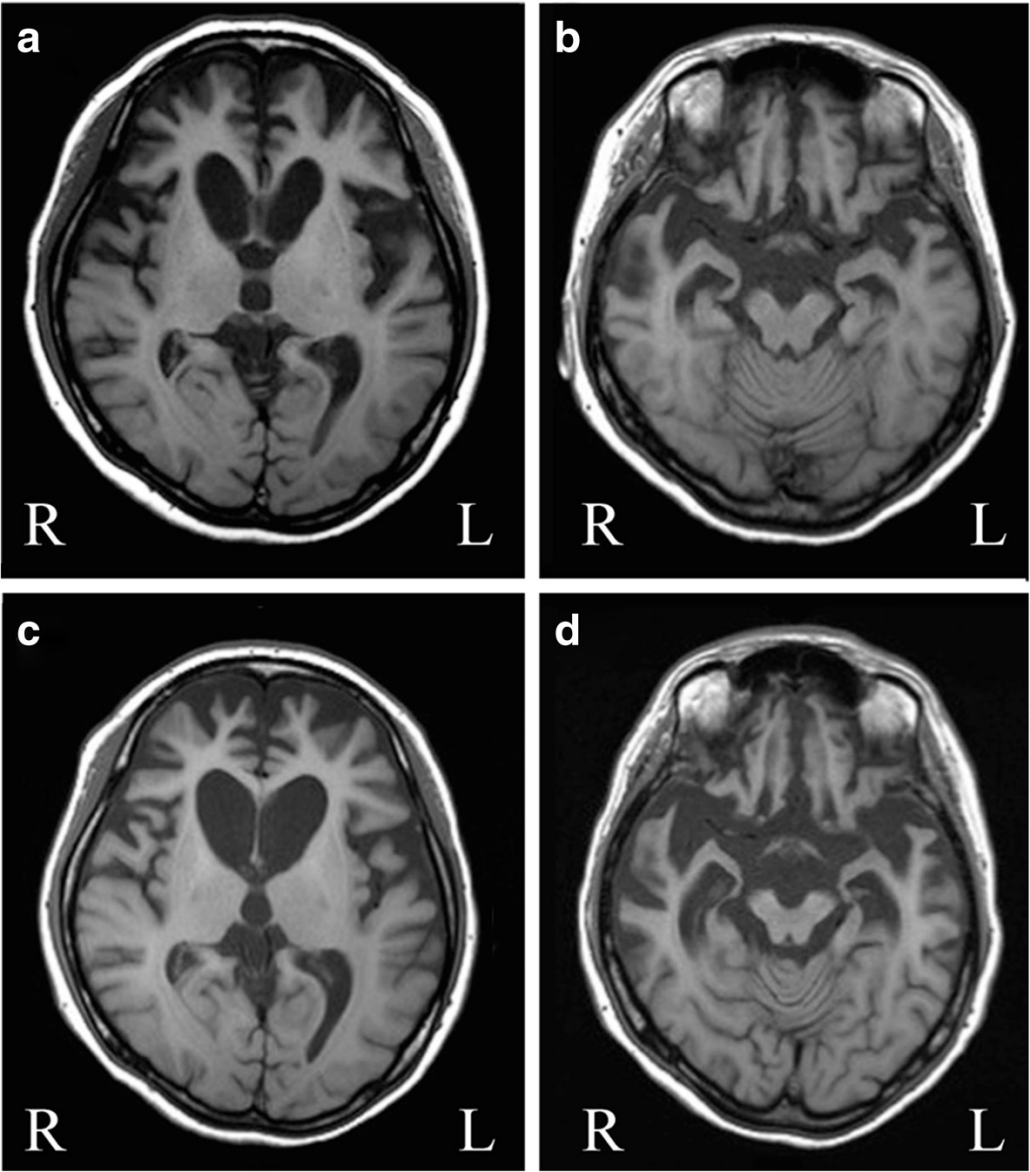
Brain MRI of case 1. a and b Brain magnetic resonance (MR) images of the patient 1 at age 36. Atrophy is present in the frontal and temporal cortices and the caudate nucleus. a and b Brain MR images of the patient taken 1 year after those shown in c and d. The atrophy is more severe and the anterior horn of the lateral ventricles is markedly enlarged. Permission was obtained from the right holder [25]
